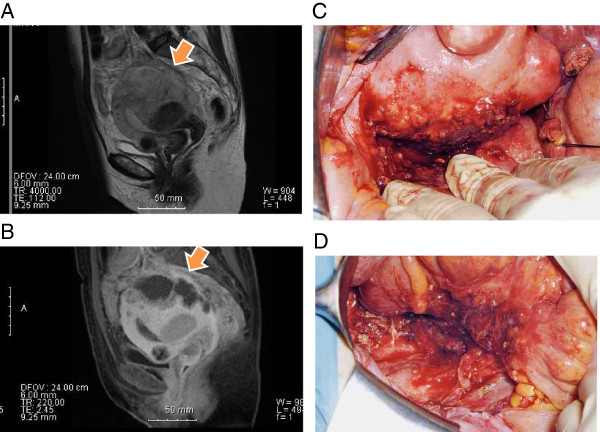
Magnetic resonance imaging (MRI) and photographs during surgery. MRI findings of a 6 cm mass in diameter (arrow) with high-intensity by sagittal T2-weighted (A) and low-intensity by sagittal T1-weighted (fat-suppressed) imaging, combined with gadolinium-enhanced imaging (B), located in the posterior myometrium. (C, D) Intra-pelvic adhesions secondary to perimetrial inflammation. Adhesions around the posterior wall of the uterus (C) and sigmoid colon (D).
